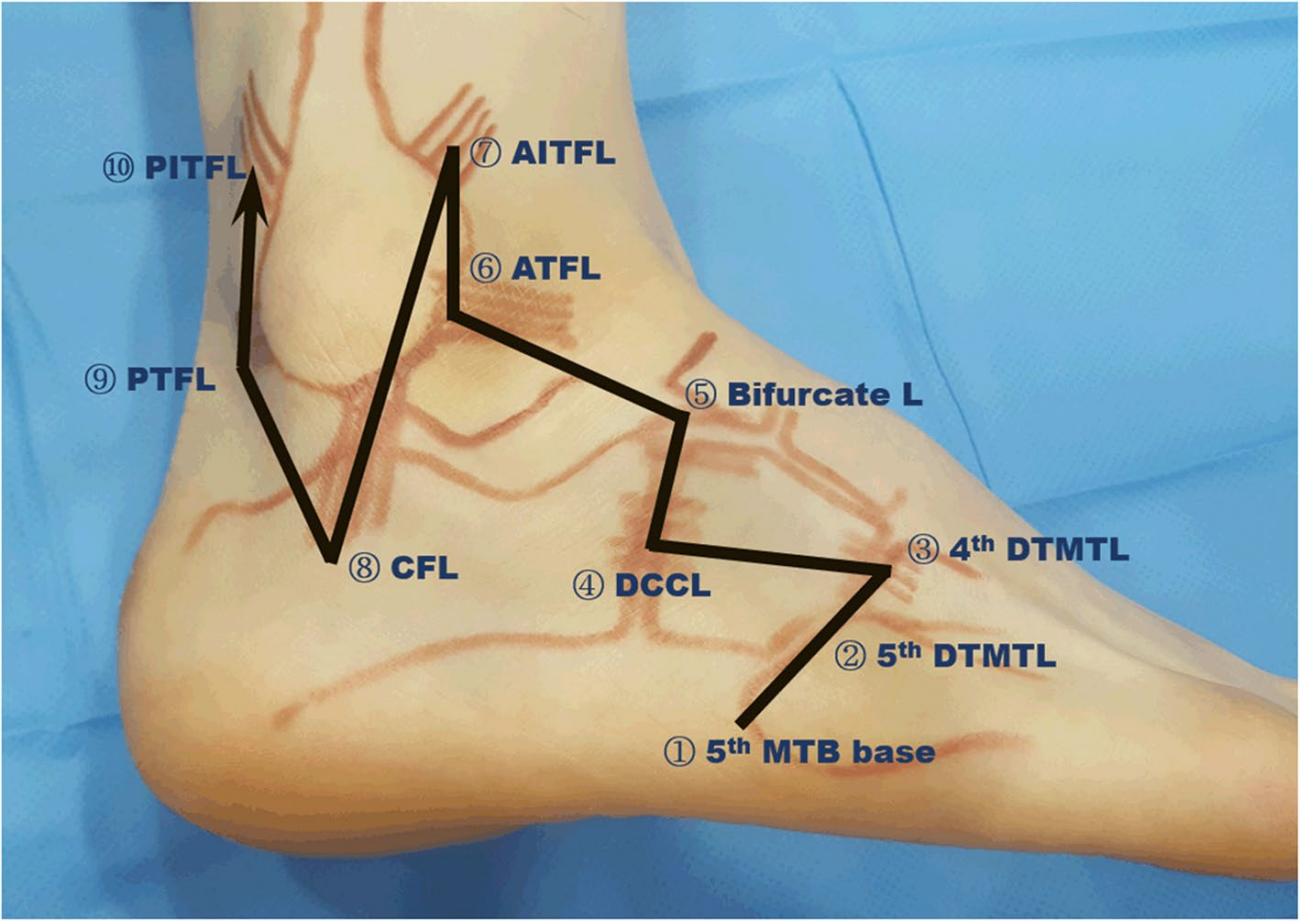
Surface anatomy and ultrasound examination of ankle. Ultrasound examination was performed in the order of the indicated numbers. 5th MTB, fifth metatarsal bone; 5^th^ and 4^th^ DTMTLs, fifth and fourth dorsal tarsometatarsal ligaments; DCCL, dorsal calcaneocuboid ligament; L, ligament; ATFL, anterior talofibular ligament; AITFL, anterior inferior tibiofibular ligament; CFL, calcaneofibular ligament; PTFL, posterior talofibular ligament; PITFL, posterior inferior tibiofibular ligament
